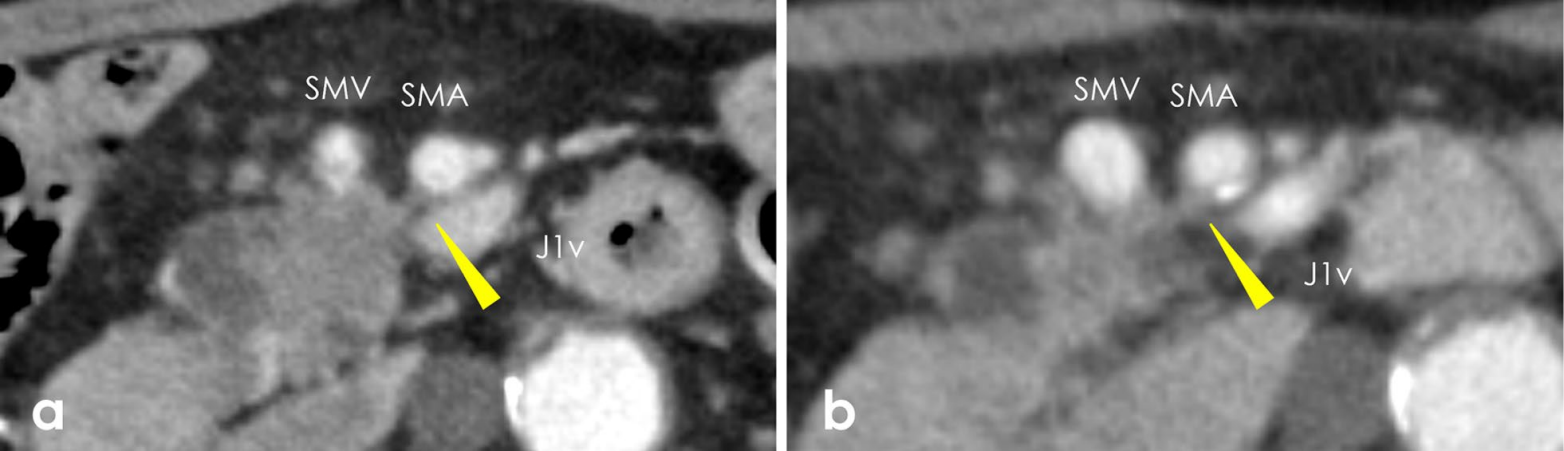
Abdominal contrast-enhanced computed tomography: a before neoadjuvant chemotherapy and b after neoadjuvant chemotherapy. Arrowheads indicate ≤ 180° of tumor involvement with the superior mesenteric artery. a A 24-mm low-density mass was detected at the uncinate process of the pancreas. The tumor involved ≤ 180° of the circumference of the superior mesenteric vein and artery. b The tumor shrank to 19 mm in size, and the response to preoperative chemotherapy was stable disease. The classification of tumor resectability was unchanged
